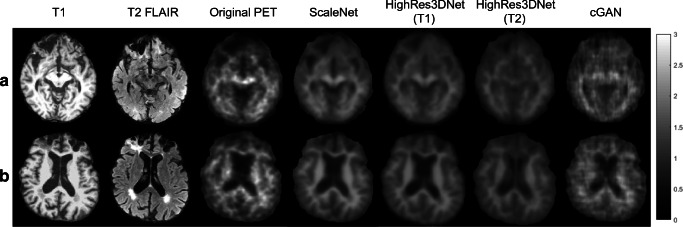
T1-weighted, T2-weighted FLAIR, and original PET images of a patient with (a) stroke and (b) WMH, with the corresponding NS images estimated using HighRes3DNet (T1-weighted and T2-weighted FLAIR input separately), ScaleNet, and cGAN (T2-weighted FLAIR as input). The PET and estimated NS images were set to the same image intensity range
